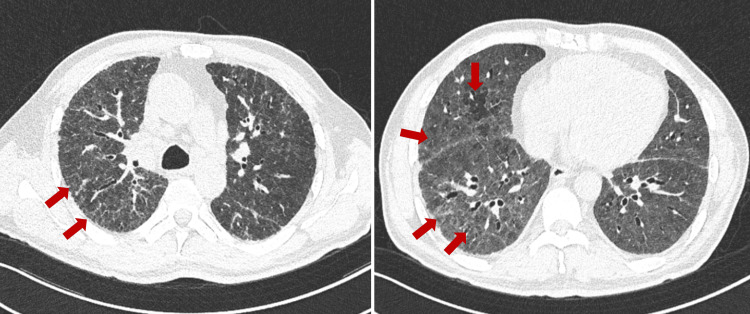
Chest CT on admission Pulmonary fibrosis (reticular opacities and traction bronchiectasis due to fibrosis formation) and headcheese sign (ground-glass opacities, normally ventilated lung tissue, and areas of air trapping). CT: computed tomography
